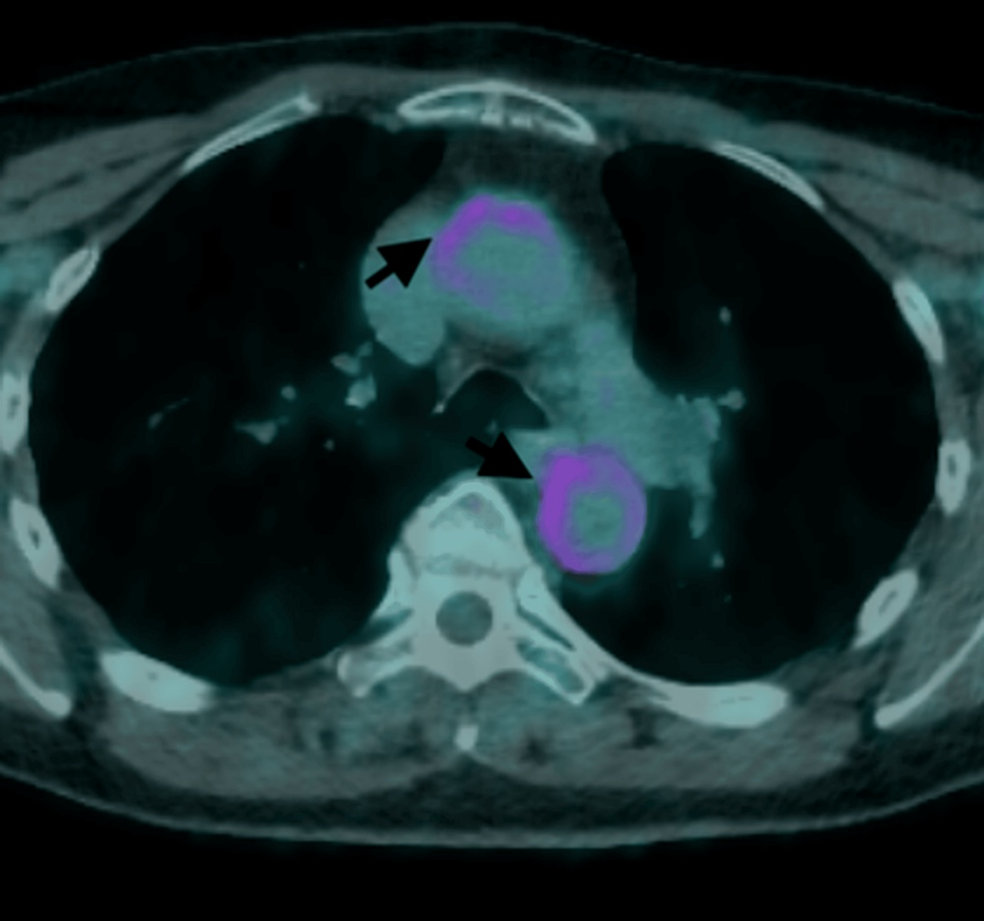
FDG-PET scan showing enhanced FDG activity in both the ascending and descending aortas (black arrows) FDG-PET: 18F-fluorodeoxyglucose-positron emission tomography
